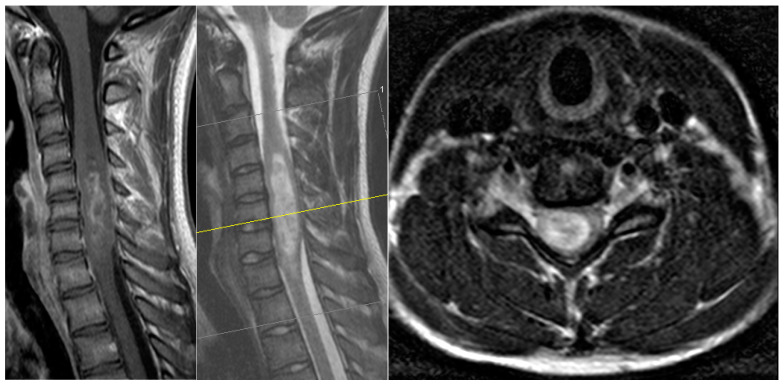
Magnetic resonance images showing the preoperative MRI scan of the patient with glioblastoma of the spinal cord at C4-Th1 (T2 and T1 with contrast enhancement, 1: sagittal view).
